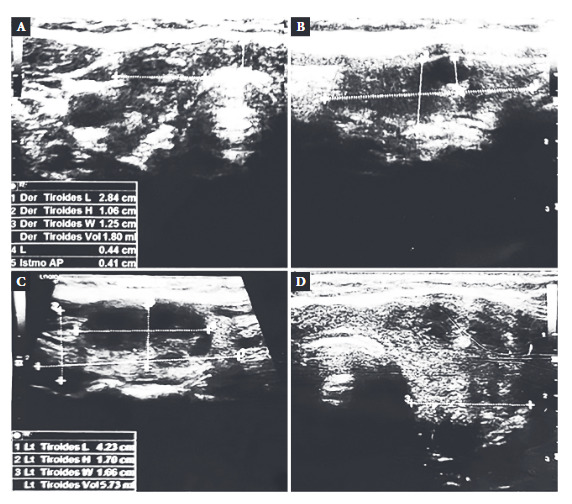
Thyroid ultrasound: (A) Right thyroid lobe, longitudinal and (B) transverse sections show a heterogeneous parenchyma with hypoechogenic areas with poorly defined borders. (C) Left thyroid lobe, longitudinal section shows a predominantly hypoechogenic heterogeneous nodule of 2.78 cm (longitudinal diameter) x 1.5 cm (transverse diameter). (D) Cross section, there is an increase in volume and heterogeneous parenchyma with some poorly defined hypoechogenic areas.
